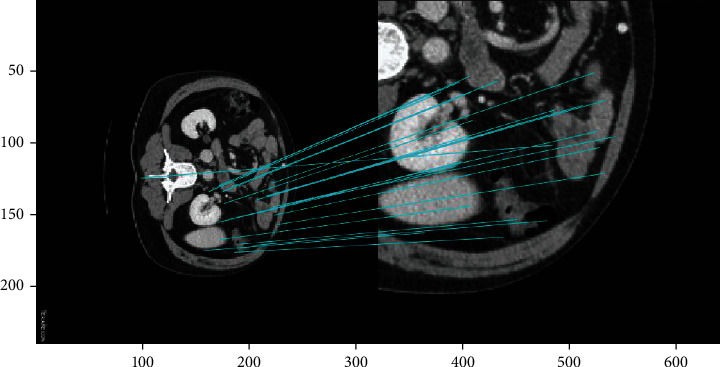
Image registration of the edge area and the whole area of CT images of human abdominal fat.
